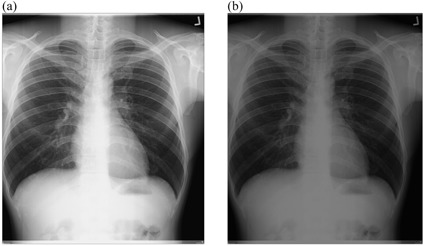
High kVp chest radiograph: (a) original image and (b) processed image by linear spatial filtering alone.
